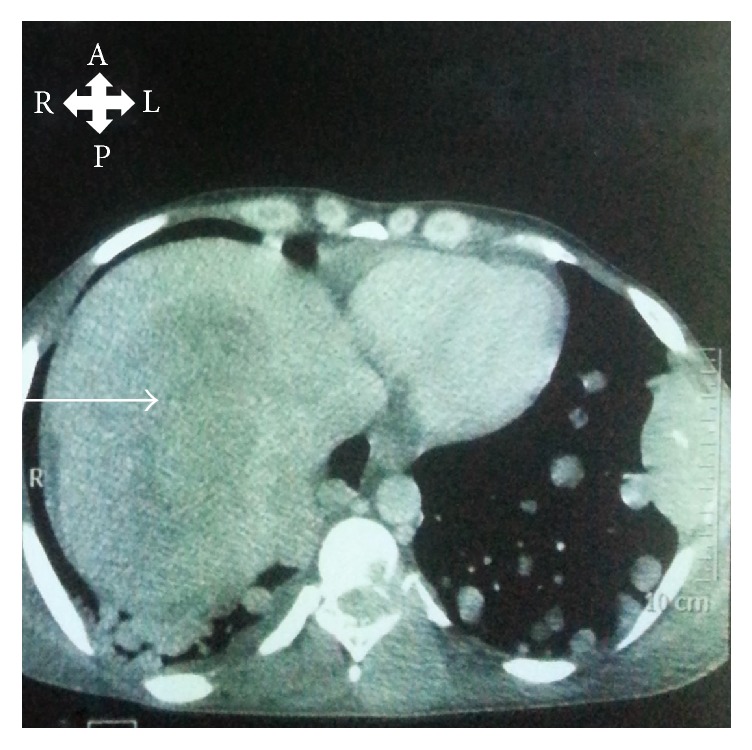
Thoracoabdominal CT scan (axial view) showing large heterogeneous and partially necrotic liver mass of 181 × 140 mm located in the right hepatic lobe (white arrow). A (anterior), L (left), R (right), and P (posterior).
